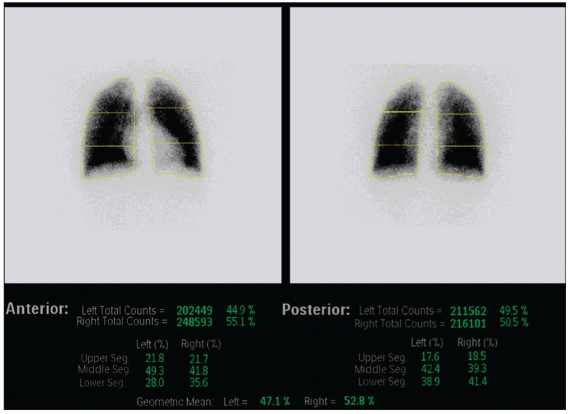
Normal quantitative lung perfusion scintigraphy (LPS) in a 16-year-old male with transposition of great arteries and S/P arterial switch operation. Quantitative perfusion analysis reveals that the right lung contributes to 53% and the left lung contributes to 47% of the total pulmonary perfusion
